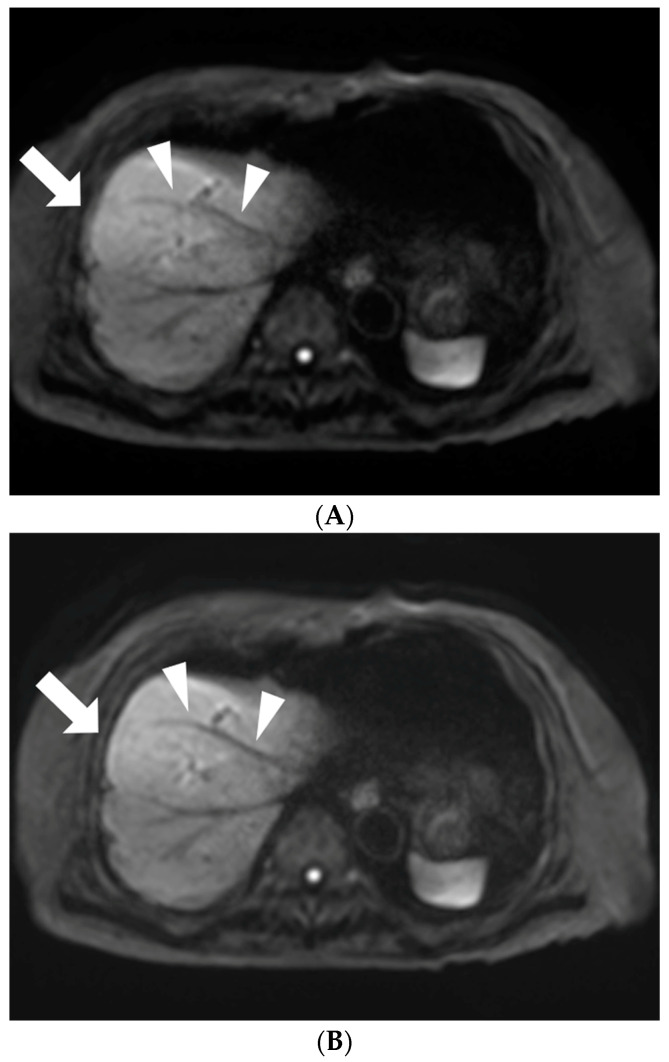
An 81-year-old woman with gallbladder cancer who underwent DWI. (A) Axial c-DWI (b = 800 s/mm^2^) and (B) axial moco-DWI (b = 800 s/mm^2^). The delineation of the intrahepatic vessel (arrowheads) as well as liver margin sharpness (arrows) are superior in moco-DWI (B) to those in c-DWI (A).
